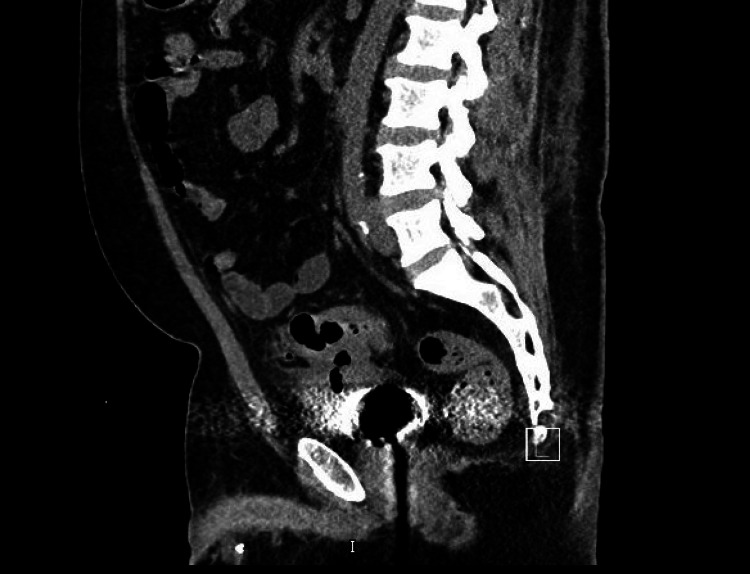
Repeat CT of the abdomen and pelvis Gas is seen in the urinary bladder, raising suspicion for a CVF CVF: colovesical fistula
